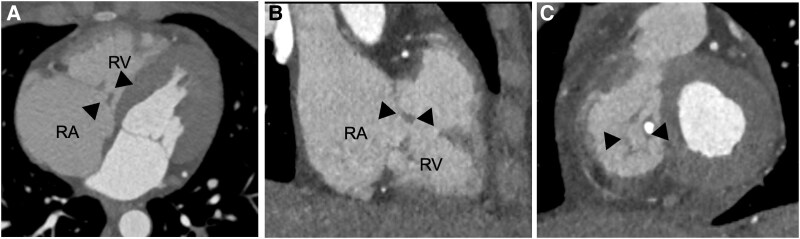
Cardiac computed tomography in four-chamber view (*A*), two-chamber view (*B*), and short-axis view (*C*). Dilation of the right-sided chambers, including the right atrium and right ventricle, is noted (*A*). The arrows indicate significant thickening of all three leaflets, with calcification of the septal leaflet and central coaptation defect. RA, right atrium; RV, right ventricle.
